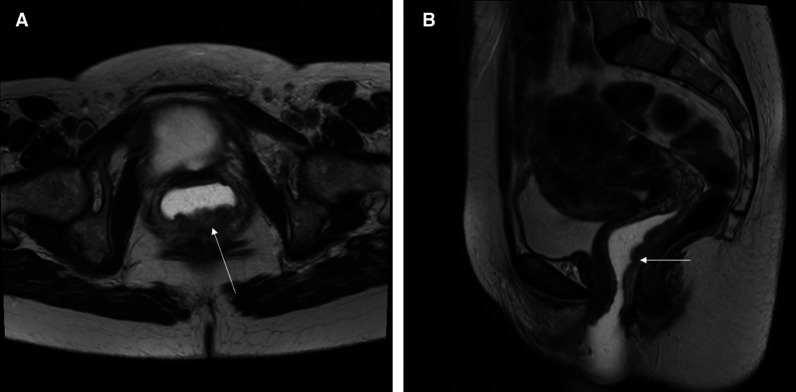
This is a T2 weighted MRI of a patient with vaginal cancer. The lesion shown by the arrow in the posterior wall of the vagina is biopsy-positive vaginal cancer. The lesion involves the entire length of the posterior vaginal wall up to 0.5 cm from the cervix. There is vaginal water base gel in the vagina which shows up as white and separates the vaginal walls so that the lesion can be seen easier.
